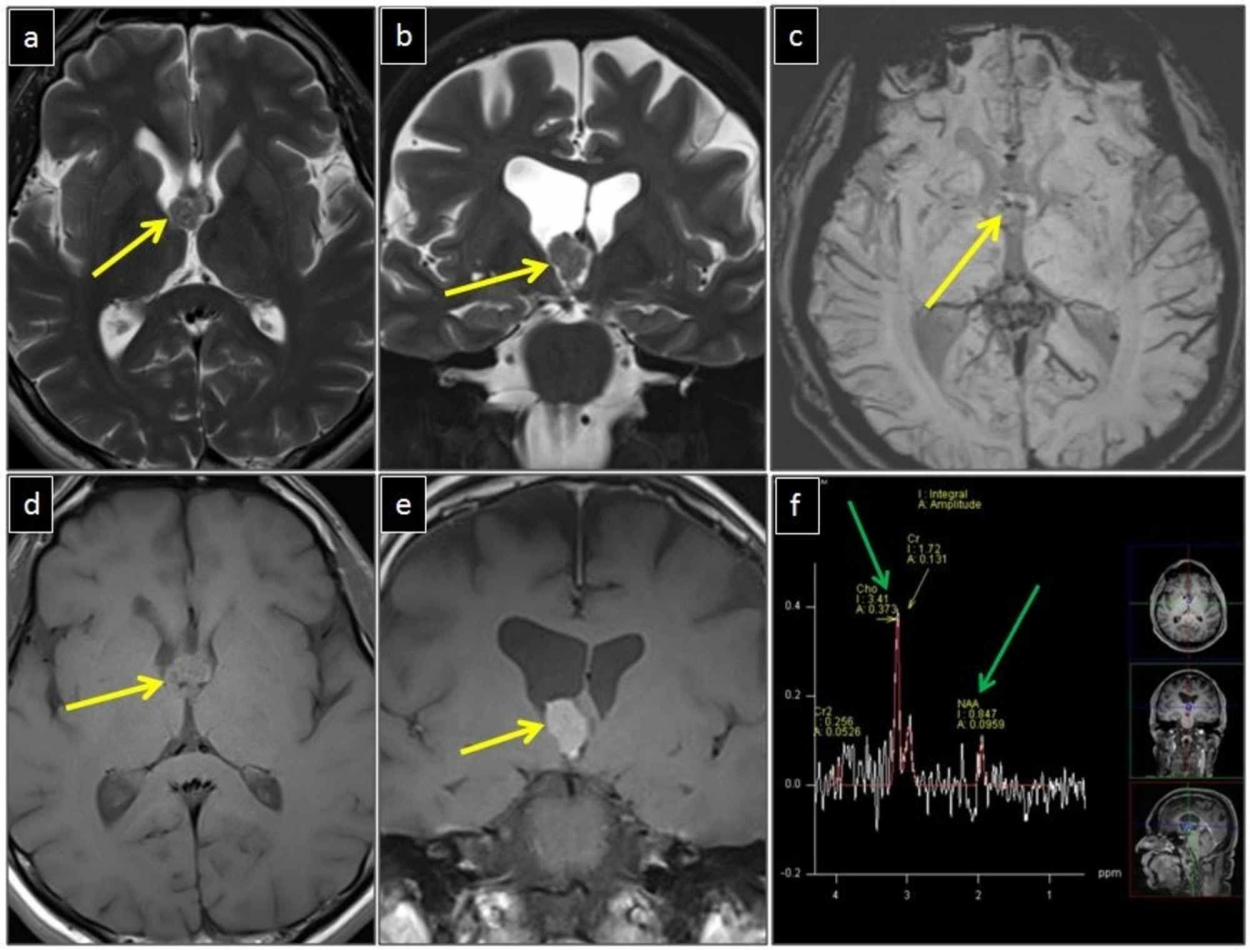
MRI brain The lesion (yellow arrows in a, b, d, e) in the anterior third ventricle has a lobulated outline. It is of intermediate signal on axial and coronal T2WI (a, b) and intermediate signal on axial T1WI (d). It shows intense homogeneous enhancement on coronal post-contrast images (e).  A few internal dark foci of blooming present on SWI (yellow arrow in c). MRS shows an increased Cho/NAA ratio within the mass (green arrows in f). No parenchymal extension or edema is seen
